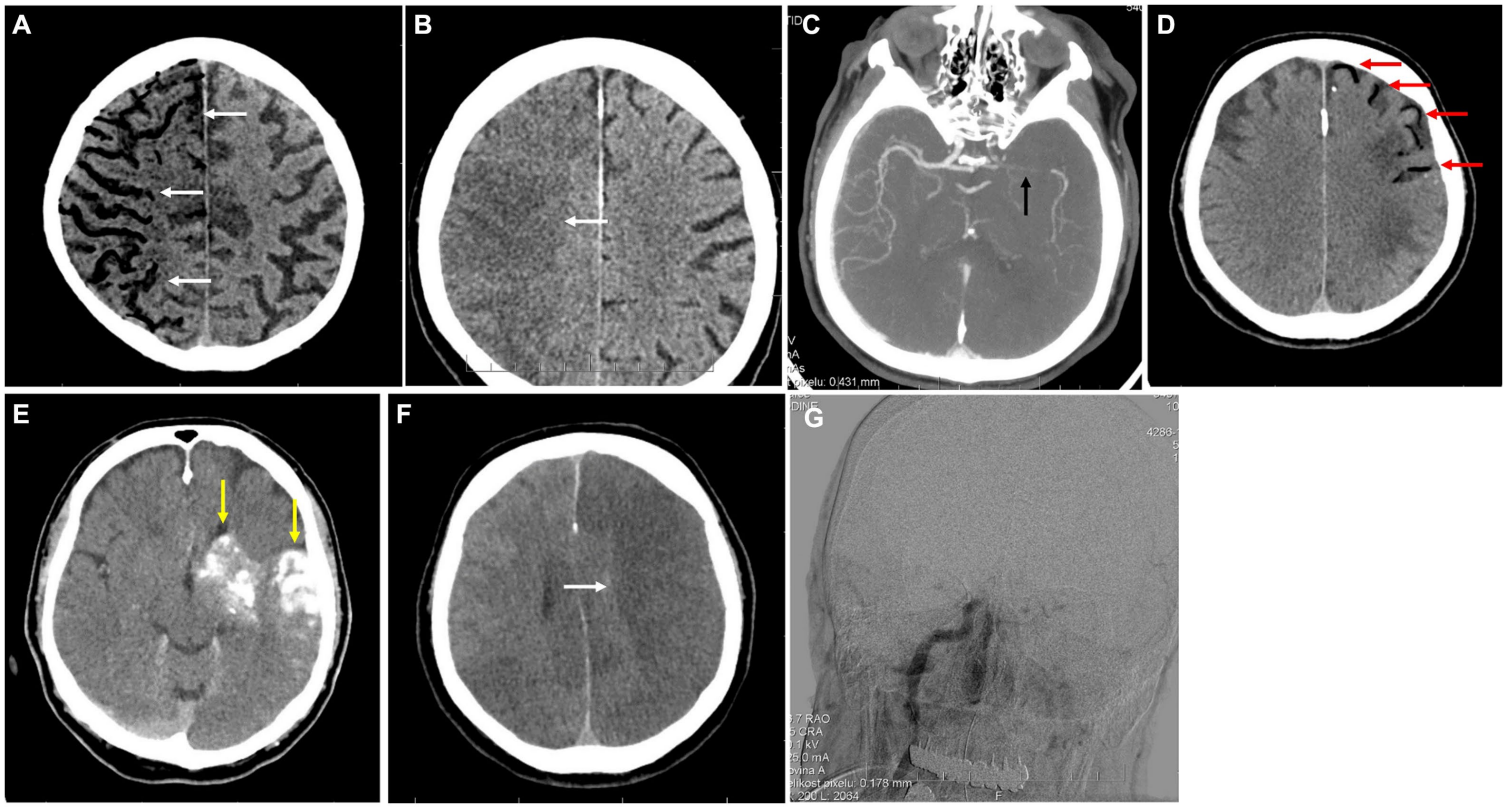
Imaging for cases 4 (A,B) and 5 (C–G). (A) Axial native CT scan of the brain in MinIP shows a very significant amount of air bubbles in the cerebral circulation in the right hemisphere (white arrows). (B) Axial CT scan of the brain reveals extensive delineated ischemia of almost the entire right cerebral hemisphere (white arrow). (C) Axial CTA of the brain showing occlusion of the M1 segment of the left middle cerebral artery (black arrow). (D) Axial native CT scan with a significant volume of air in the brain circulation on the left side (red arrows). (E) Native axial CT scan with contrast agent extravasation and intracerebral and subarachnoid hemorrhage (yellow arrows). (F) Axial native CT scan of the brain with developing extensive acute ischemia in the left middle cerebral artery territory (white arrow). (G) Cerebral panangiography without intracranial arterial flow, demonstrating brain death.
